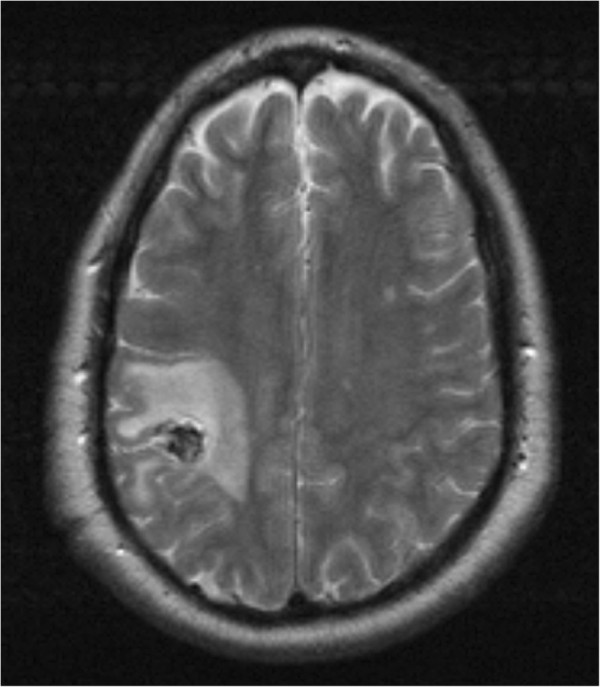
Axial T2-weighted image demonstrating a popcorn-shaped predominantly hypointense lesion in the parietal lobe with perifocal edema.
